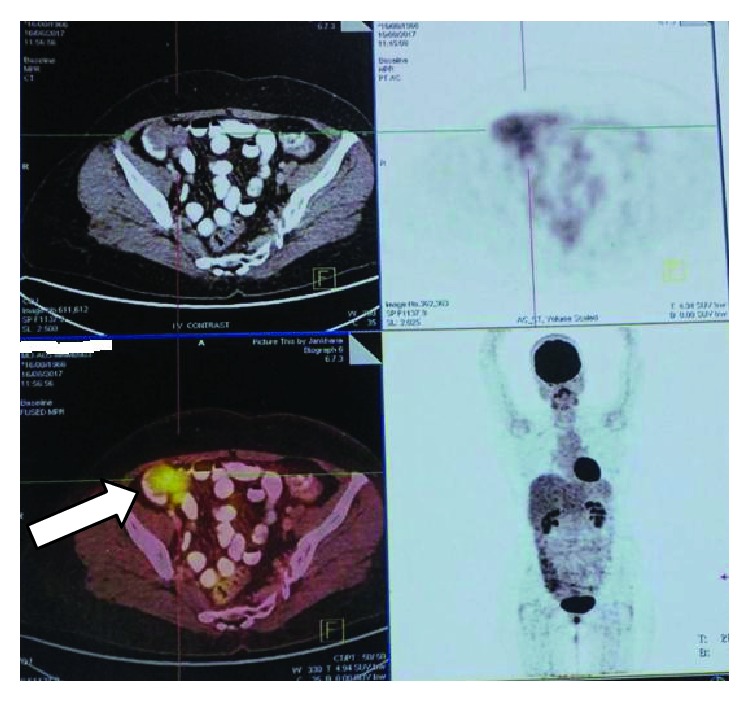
PET/CT scan showing well-defined heterogeneously enhancing lesion in the right iliac fossa along the anterior abdominal wall.
